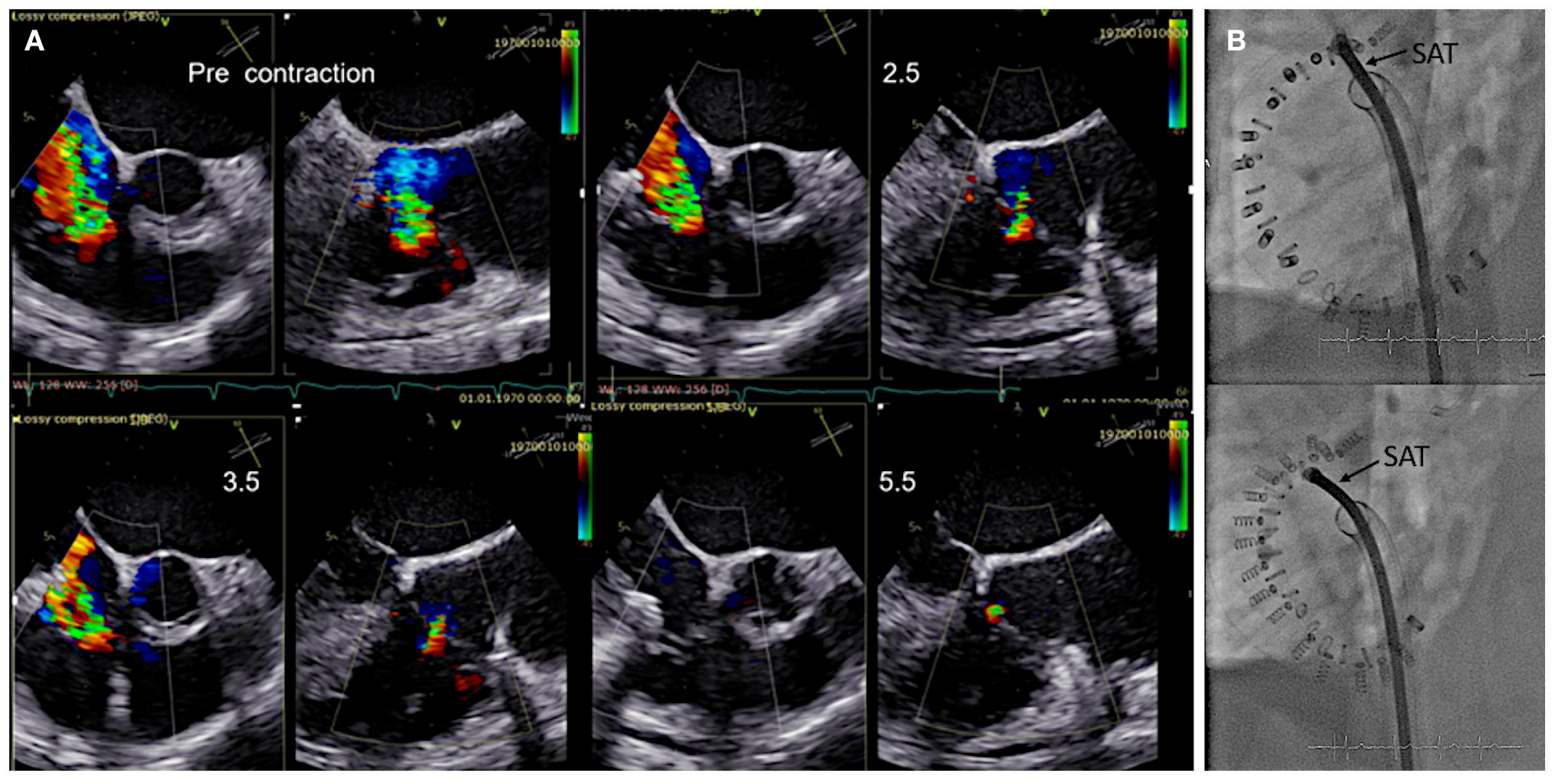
Monitoring during step-wise contraction of the implant. (A) Evaluation of the reduction of tricuspid regurgitation (TR) under gradual increasing contraction (2.5, 3.5, and 5.5 cm) are shown in x-plane imaging with color Doppler (TEE 60? left/150? right). Under maximum contraction (5.5 cm; bottom right) only trivial residual TR is visible. (B) The implant size and shape is seen in fluoroscopy before (upper image) and after (lower image) contraction. SAT, size adjustment tool.
